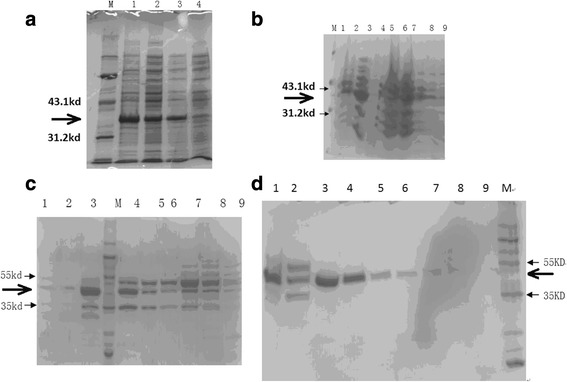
Expression and purification of SFRP5 protein. a Identification of pET30-SFRP5 expression. M marker (97.4kd,66.2kd,43.1kd,31.2kd,21.2kd,14.4kd). 1. Precipitate of ultrasound-disrupted *E. coli* cells induced by pET30-SFRP5 for 4 h; 2. Supernatant of ultrasound-disrupted *E. coli* cells induced by pET30-SFRP5 for 4 h; 3. Ultrasound-disrupted *E. coli* cells induced by pET30-SFRP5 for 4 h; 4. Ultrasound-disrupted *E. coli* cells. b The supernatant of the disrupted bacteria was subjected to hydrophobic chromatography, and the bacterial supernatant protein was added to 2 M ammonium sulfate, followed by elution. M marker (97.4kd,66.2kd,43.1kd,31.2kd,21.2kd,14.4kd). 1-9: elusion products. c The hydrophobic chromatography eluate was titrated to PH 8.0 and subjected to ion exchange chromatography. 1. DEAE sample before loading; 2. DEAE penetration sample; 3. 20 mM PB 50 mM NaCl PH 8.0 eluent 1; M:Marker (130kd,100kd,70kd,55kd,35kd,25kd,15kd,10kd); 4: 20 mM PB 50 mM NaCl PH 8.0 eluent 2; 5: 20 mM PB 50 mM NaCl PH 8.0 eluent 3; 6: 20 mM PB 50 mM NaCl PH 8.0 eluent 4; 7: 20 mM PB 100 mM NaCl PH 8.0 eluent 1; 8: 20 mM PB 100 mM NaCl PH 8.0 eluent 2; 9: 20 mM PB 100 mM NaCl PH 8.0 eluent 3. d Affinity chromatography: 20 mM PB 50 mM NaCl 8.0 pH 8.0 ion exchange chromatography eluate was subjected to nickel column affinity chromatography. 1. Ni sample before loading; 2. Ni penetration sample; 3. 20 mM PB 0.15 M NaCl 5 mM imidazole PH 8.0 eluent 1; M Marker (130kd,100kd,70kd,55kd,35kd,25kd,15kd,10kd); 4. 20 mM PB 0.15 M NaCl 5 mM imidazole PH 8.0 eluent 2; 5. 20 mM PB 0.15 M NaCl 5 mM imidazole PH 8.0 eluent 3; 6. 20 mM PB 0.15 M NaCl 30 mM imidazole PH 8.0 eluent 1; 7. 20 mM PB 0.15 M NaCl 30 mM imidazole PH 8.0 eluent 2; 8. 20 mM PB 0.15 M NaCl 30 mM imidazole PH 8.0 eluent 3; 9. 20 mM PB 0.15 M NaCl 30 mM imidazole PH 8.0 eluent 4
